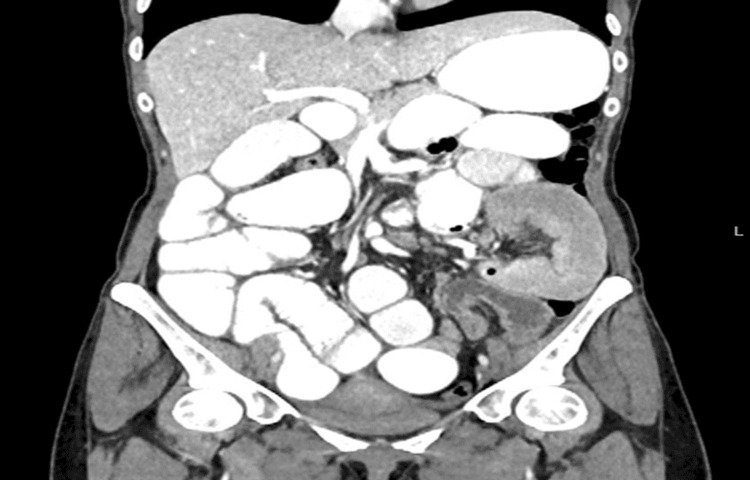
Computed tomography of the abdomen and pelvis with contrast showing dilated stomach and proximal small bowel loops with collapsed distal small bowel and colon
